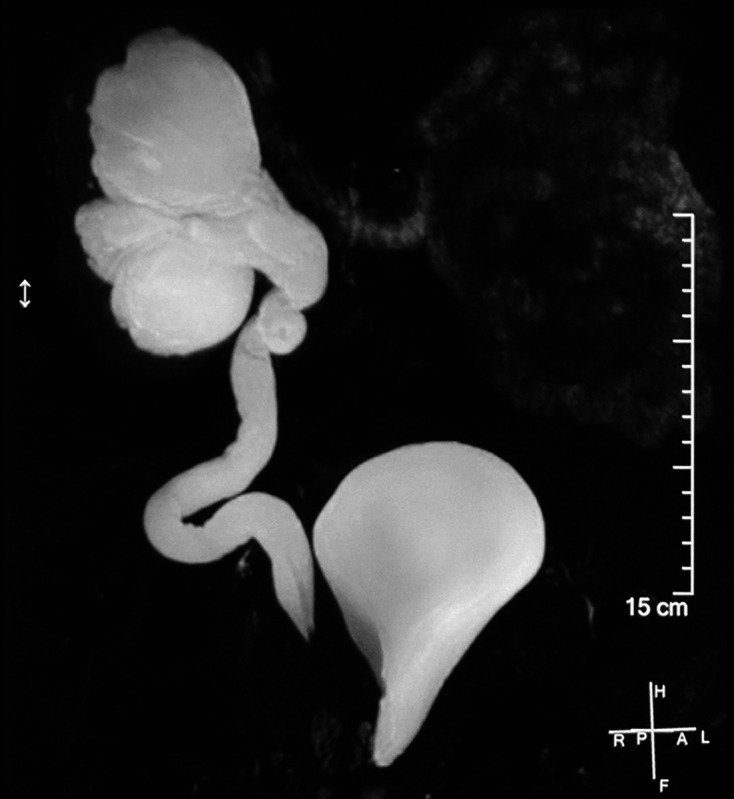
Contrast MRI showing pear-shaped bladder with right side solitary functioning kidney with gross HUN.
